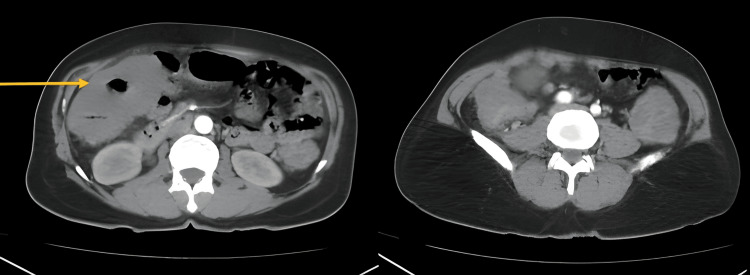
CT image showed short segment circumferential thickening of D1 and D2 was noted.
